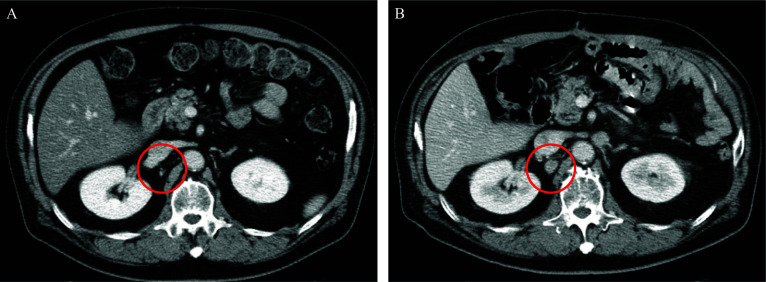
A regular follow-up contrast-enhanced computed tomography (CT) scan revealed a para-aortic lymph node recurrence (B) which was not detectable before gastrectomy (A) (red circle)
